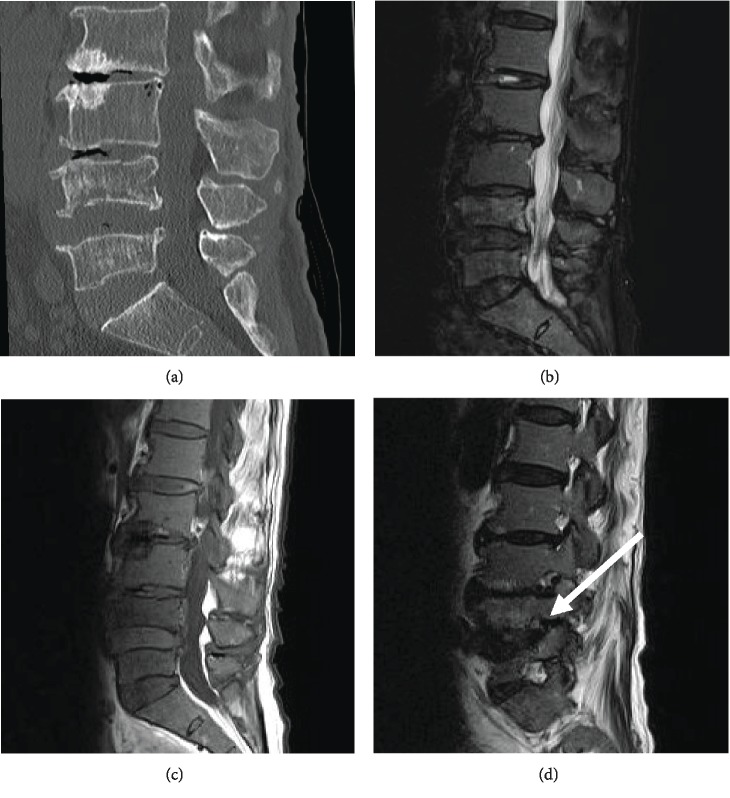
CT axial image demonstrating the decreased height of the L5 and L6 vertebral bodies (a). Sagittal magnetic resonance images showing iso- to low-intensity change in L5 vertebral body on a FLAIR (b), and low-intensity change on a T1-weighted image (c). T2 sagittal image showing compression of the left L5 nerve root at the L5/6 intervertebral foramen (d).
